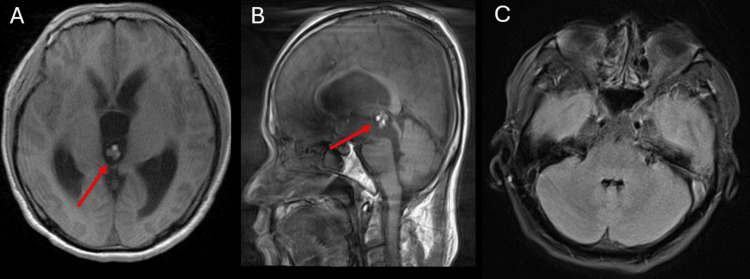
MRI of the brain with and without contrast on hospital day three. Significant motion artifact is present. A: Axial T1-weighted imaging shows ventriculomegaly and a heterogeneous cystic lesion in the third ventricle (red arrow). B: Sagittal T1-weighted imaging shows ventricular dilation and a heterogeneous cystic lesion in the third ventricle (red arrow). C: Axial T2 fluid-attenuated inversion recovery imaging shows non-dilated fourth ventricle and no evidence of cystic lesions.
